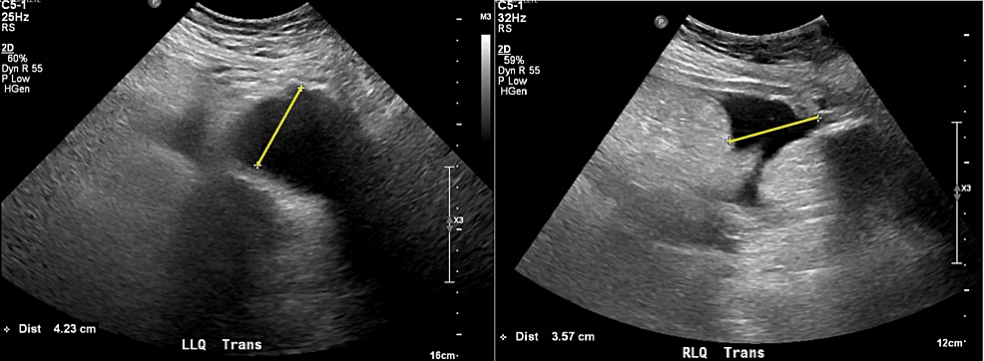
Abdominal Ultrasound for Ascites Evaluation LLQ trans: left lower quadrant transabdominal; RLQ trans: right lower quadrant transabdominal
